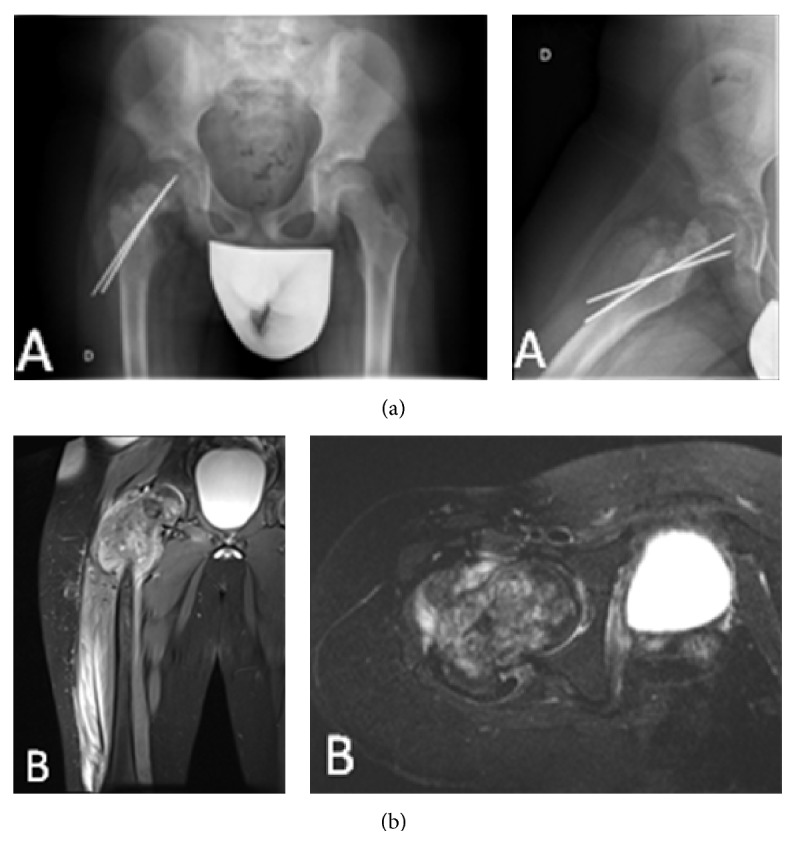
(a) Plain radiographs of the pelvis, hip, and proximal femur showing mixed osteolytic and sclerotic areas in the right proximal femur and an unhealed femoral neck fracture fixed with two K wires. (b) MRI showing a proximal femoral lesion extending distally 13 cm with a surrounding soft-tissue mass.
